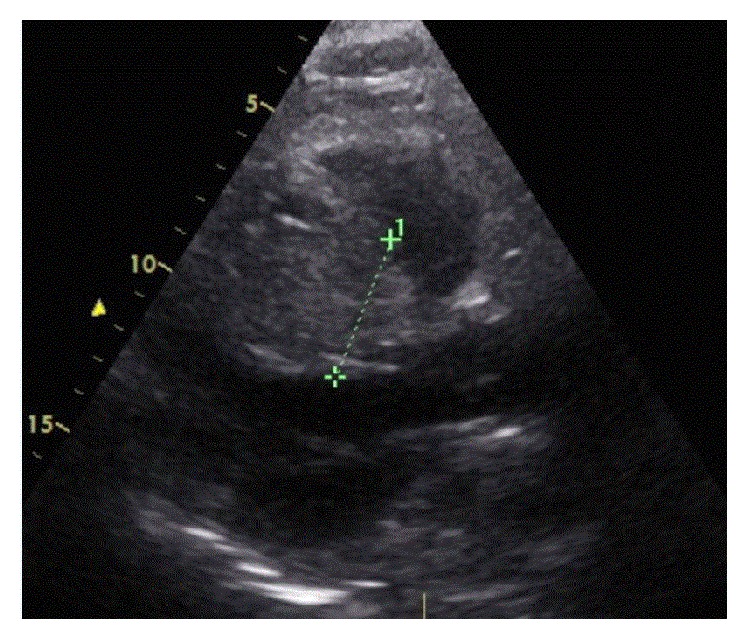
Echocardiogram shows a huge swelling of the intraventricular septal wall with compression of the right ventricle.
